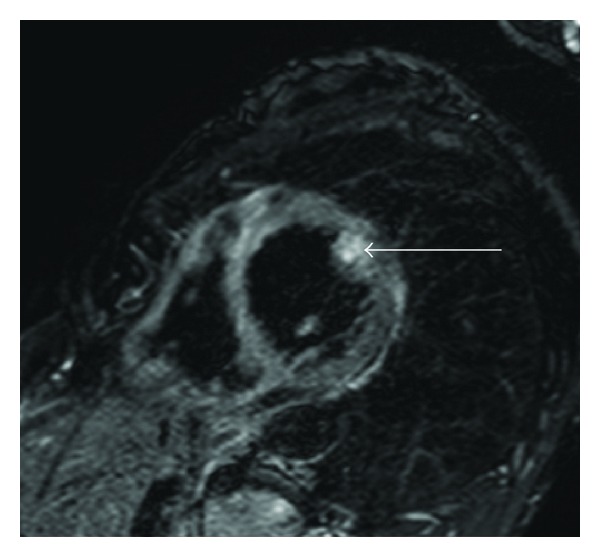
50-year-old female with Churg-Strauss syndrome. Short-axis T2-weighted MRI shows patchy myocardial edema of the papillary muscle (arrow) in the eosinophilic myocarditis associated with this syndrome.
